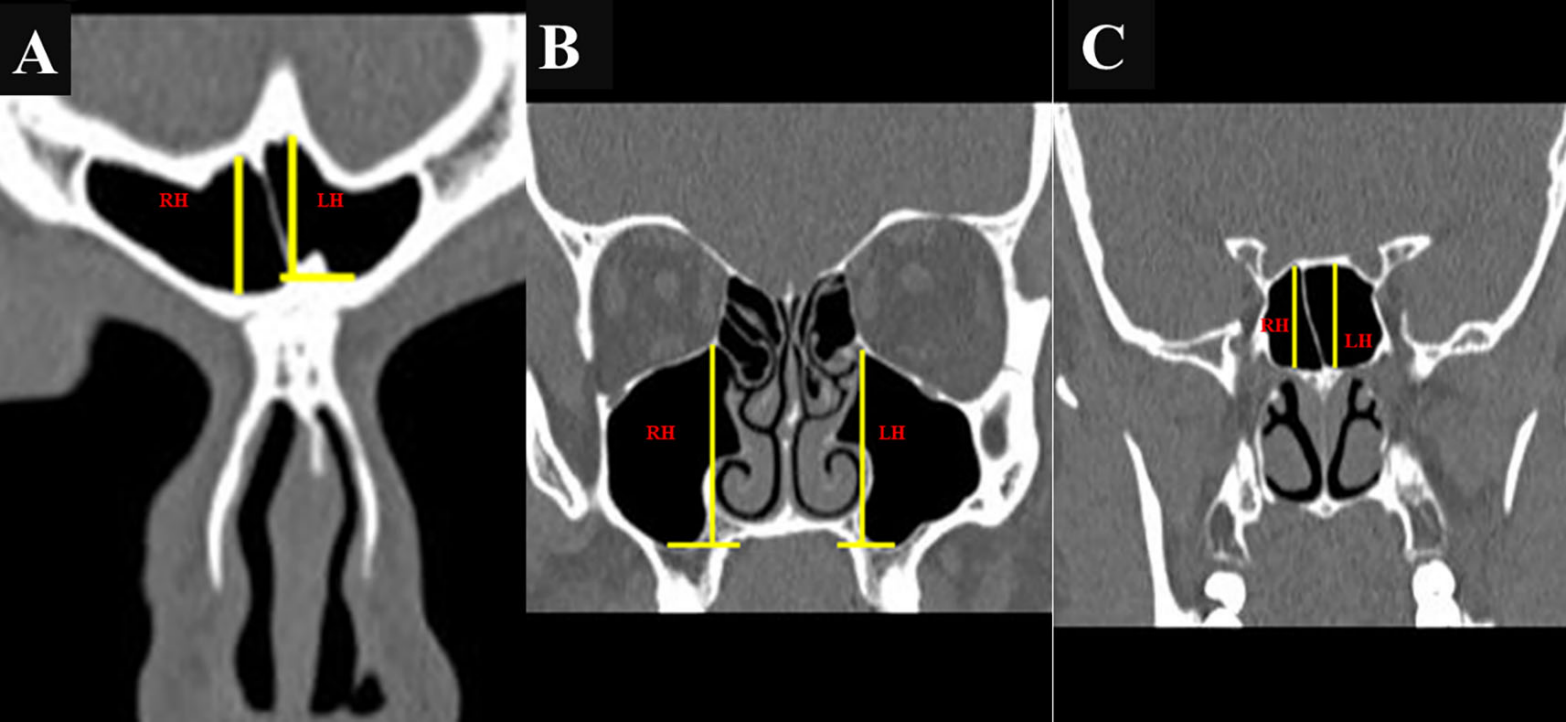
(A, B, C): Coronal CT images showing the height of FS, MS, and SS, respectively. (RH, right height; LH, left height).
